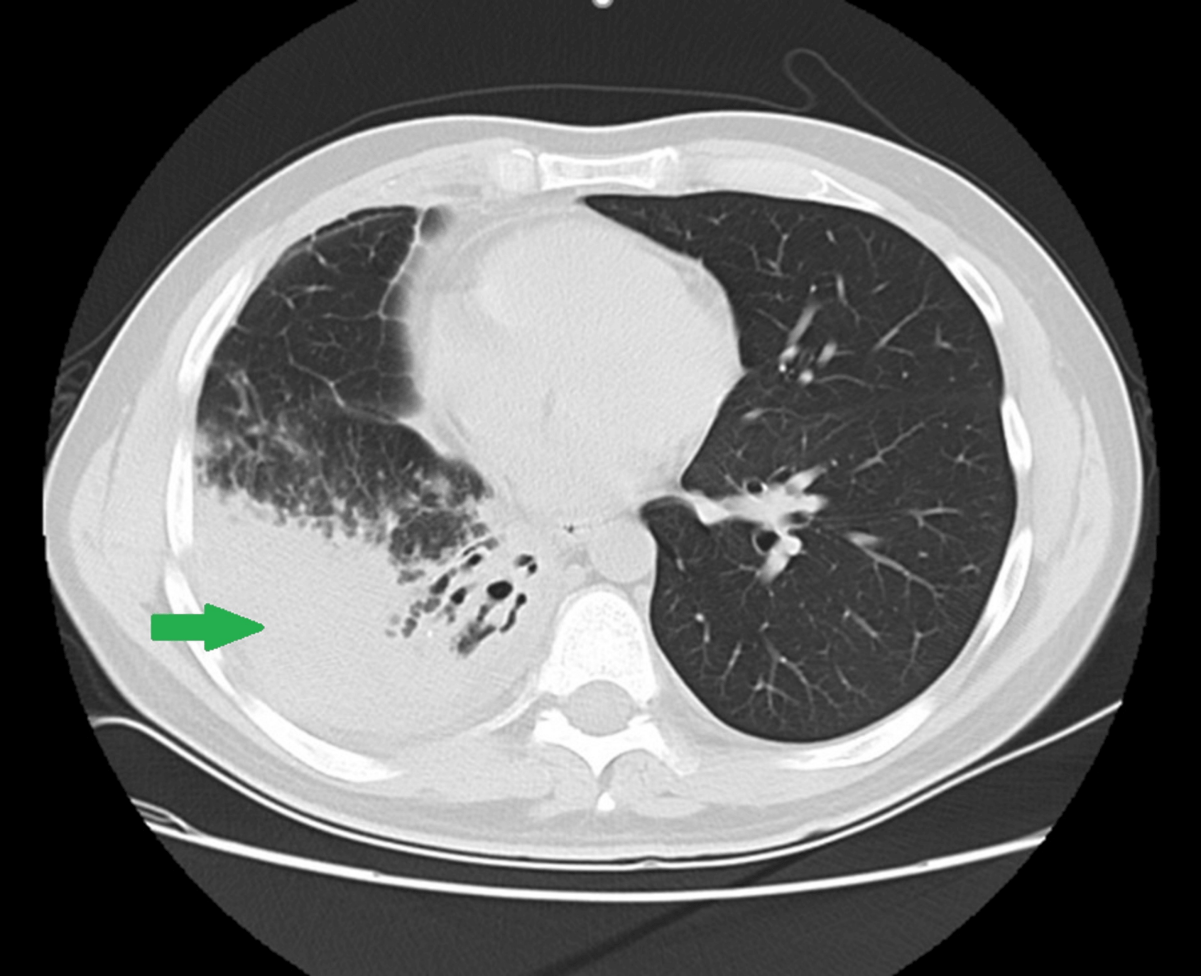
Lung CT scan showing right pleural effusion (green arrow)
